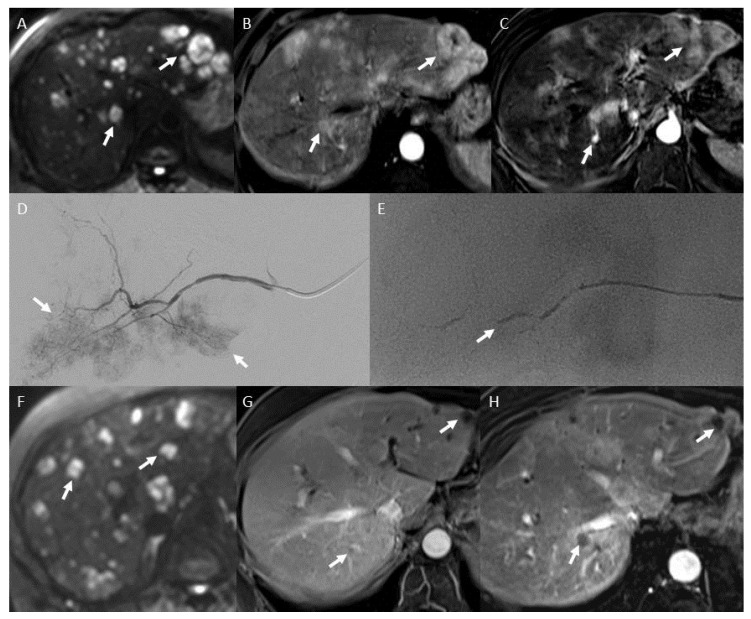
A 71-year-old woman with neuroendocrine tumor from undefined primary and liver metastases with important carcinoid syndrome and liver pain related to diffuse liver involvement. Pre-embolization diffusion-weighted magnetic resonance (MR) image in the axial plane, (A), T1-weighted MR image after intravenous administration of a gadolinium chelate during the arterial phase without (B) and with subtraction (C) show multiple, bilateral liver metastases with restricted diffusion (arrows), and hyperenhancement during the arterial phase (arrows). Patient had 6 sessions of conventional transarterial chemo-embolization under general anesthesia using a mixture of iodized oil (Lipiodol^®^) and doxorubicin and final embolization using calibrated 300–500 µm microspheres. Initial angiogram of right anterior hepatic artery shows multiple arterial tumor blush (arrows) (D) and final angiogram shows proximal occlusion of the right anterior hepatic artery (arrow) (E). Post-embolization diffusion-weighted MR image in the axial plane (F), T1-weighted MR images obtained after injection of gadolinium chelate during the arterial phase without (G) and with subtraction (H) show multiple, bilateral liver metastases with restricted diffusion (arrows), and hypointensity of most of metastases during the arterial phase (arrows).
